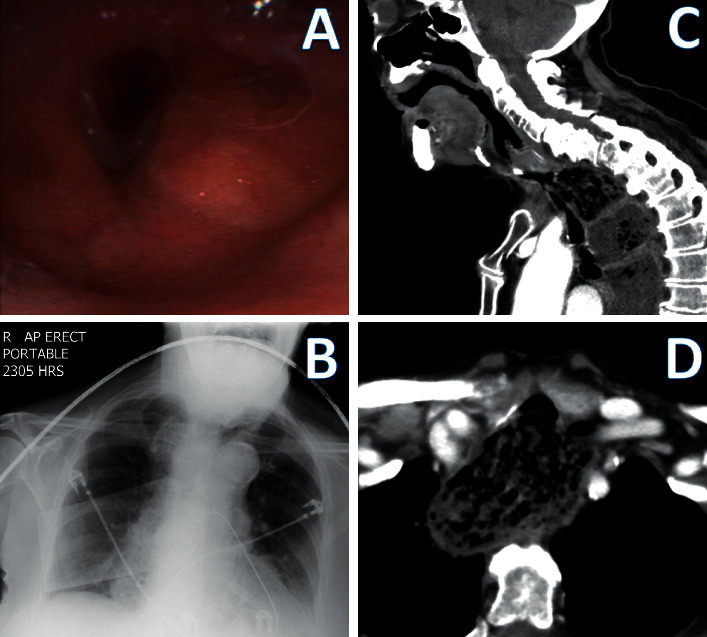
(a) Bronchoscopic image of the laryngeal inlet showing laryngeal oedema. (b) Anterior-posterior view of erect portable chest X-ray. (c) Sagittal view of CT neck showing massively dilated oesophagus and anteriorly displaced trachea and larynx. (d) Axial view of CT neck at the level of clavicles showing massively dilated oesophagus compressing the trachea anteriorly.
